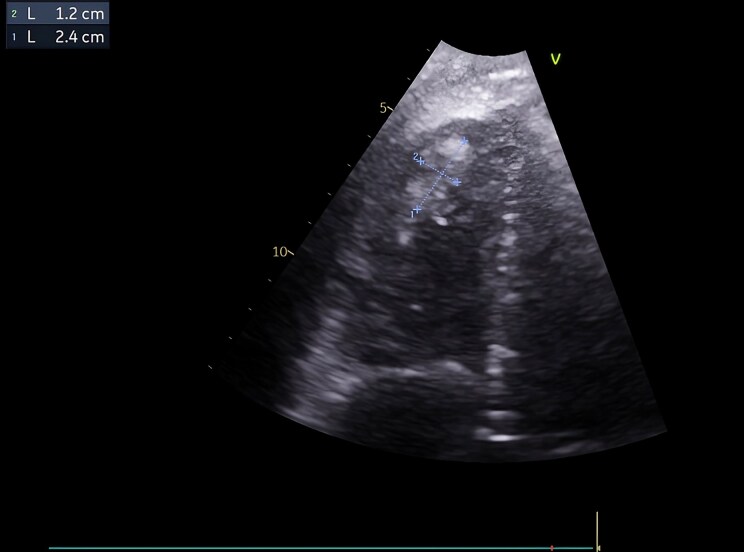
Transthoracic echocardiography, four-chamber view focused on the right ventricle, showing a mass consistent with a thrombus at the right ventricular apex.
